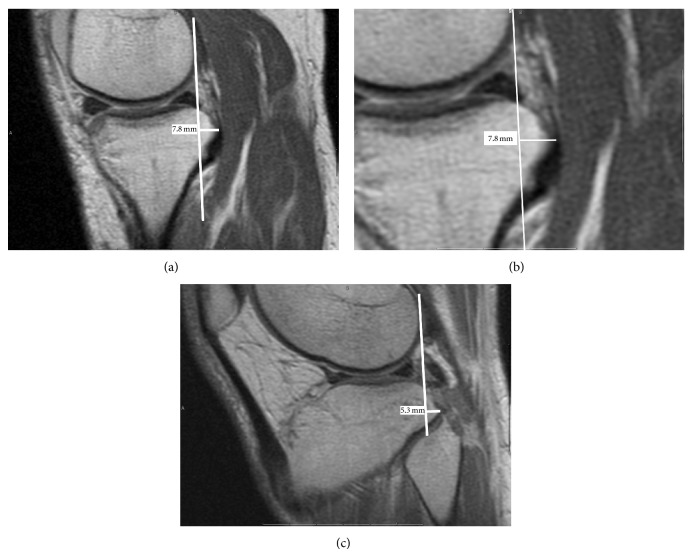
Measurement technique for tibial translation on knee MRI. Magnetic resonance images of the knee in a patient with isolated PCL tear were obtained using sagittal proton density weighted pulse sequence (TR = 3000; TE = 12.168; 1 NEX; 256 × 192 matrix; ETL = 8; 3.00 mm slice thickness). (a) Midmedial compartment measurement of tibial translation demonstrates posterior (+) tibial translation of 7.8 mm in the presence of a PCL tear. (b) Magnified view of measurement of midmedial compartment. (c) Midlateral compartment measurement of tibial translation demonstrates posterior (+) translation of 5.3 mm.
